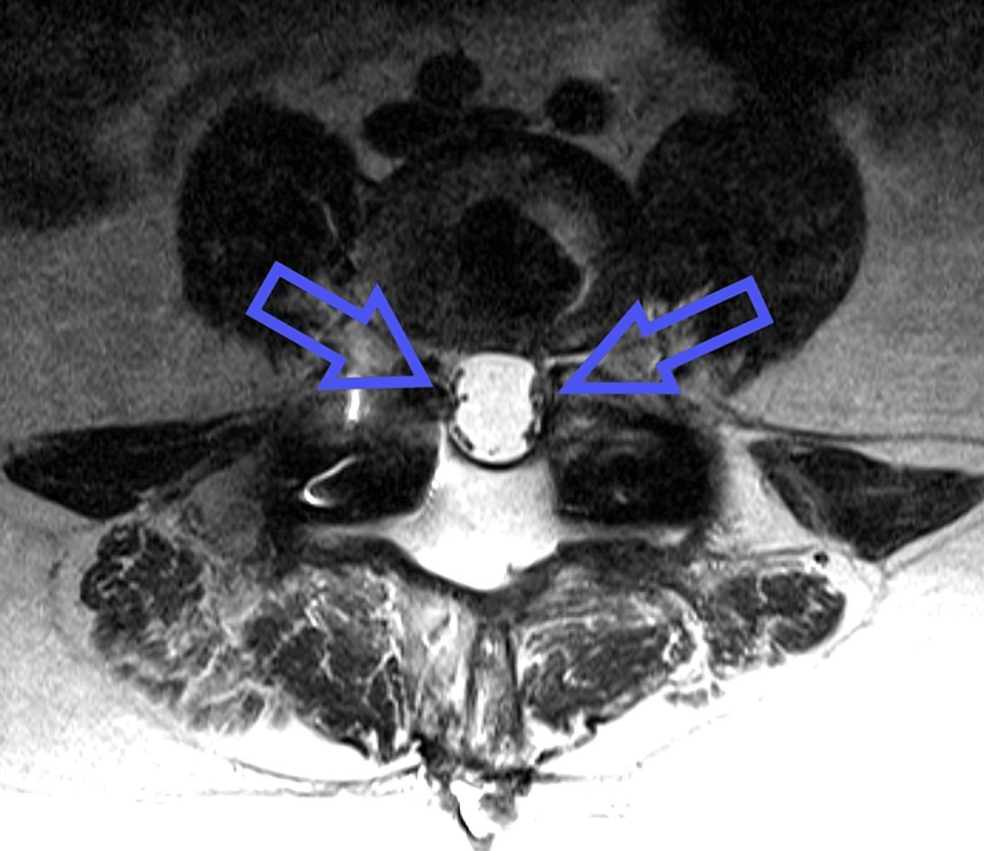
T2-weighted magnetic resonance imaging of the lumbar spine at L4-5 demonstrating clumping of the nerve roots (arrows) to the edges of the dura bilaterally (i.e., an "empty sac sign").
